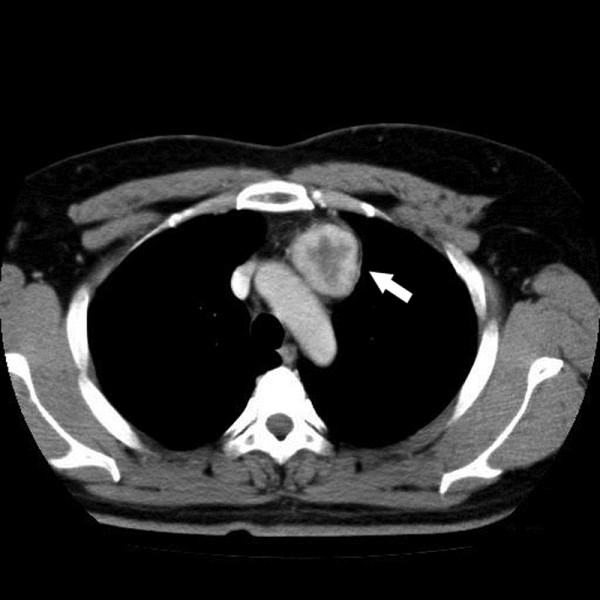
Oral contrast-enhanced CT scanning of the tumor. Oral contrast-enhanced CT revealed a well-circumscribed, solitary mass of 4.41 × 3.93 cm in the anterior superior mediastinum.
